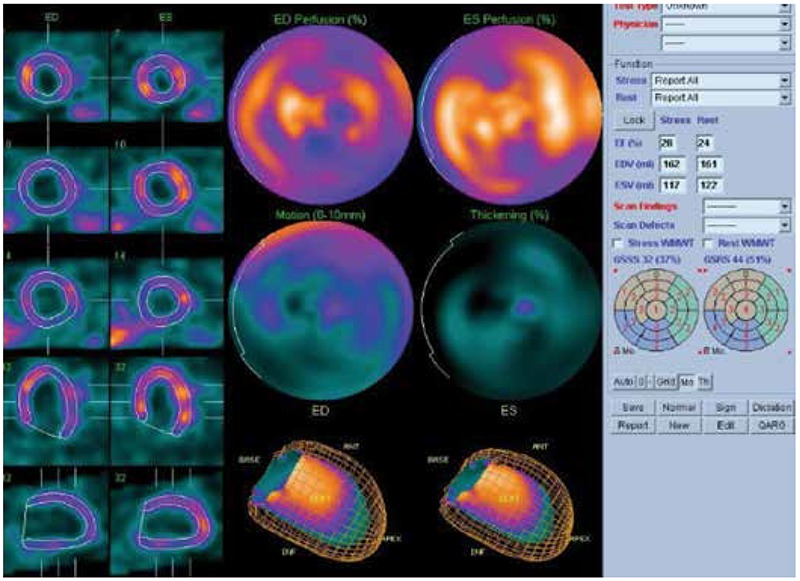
Quantitative gated single photon emission computed tomography findings of the same patient. In the GATED-single photon emission computed tomography imaging were found stress ejection fraction: 31%, resting ejection fraction: 24%
